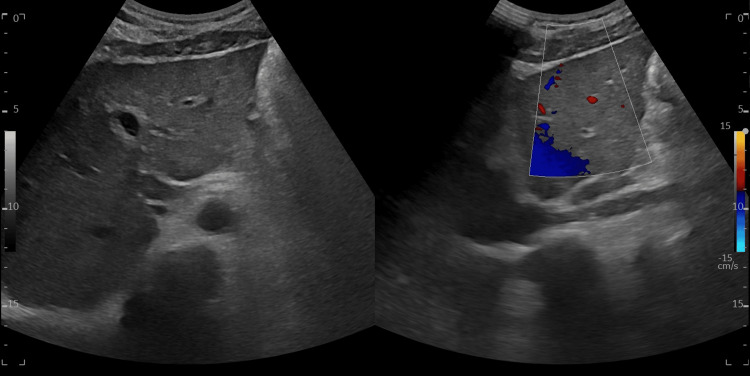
Follow-up liver ultrasound showing complete resolution of the liver abscess.
